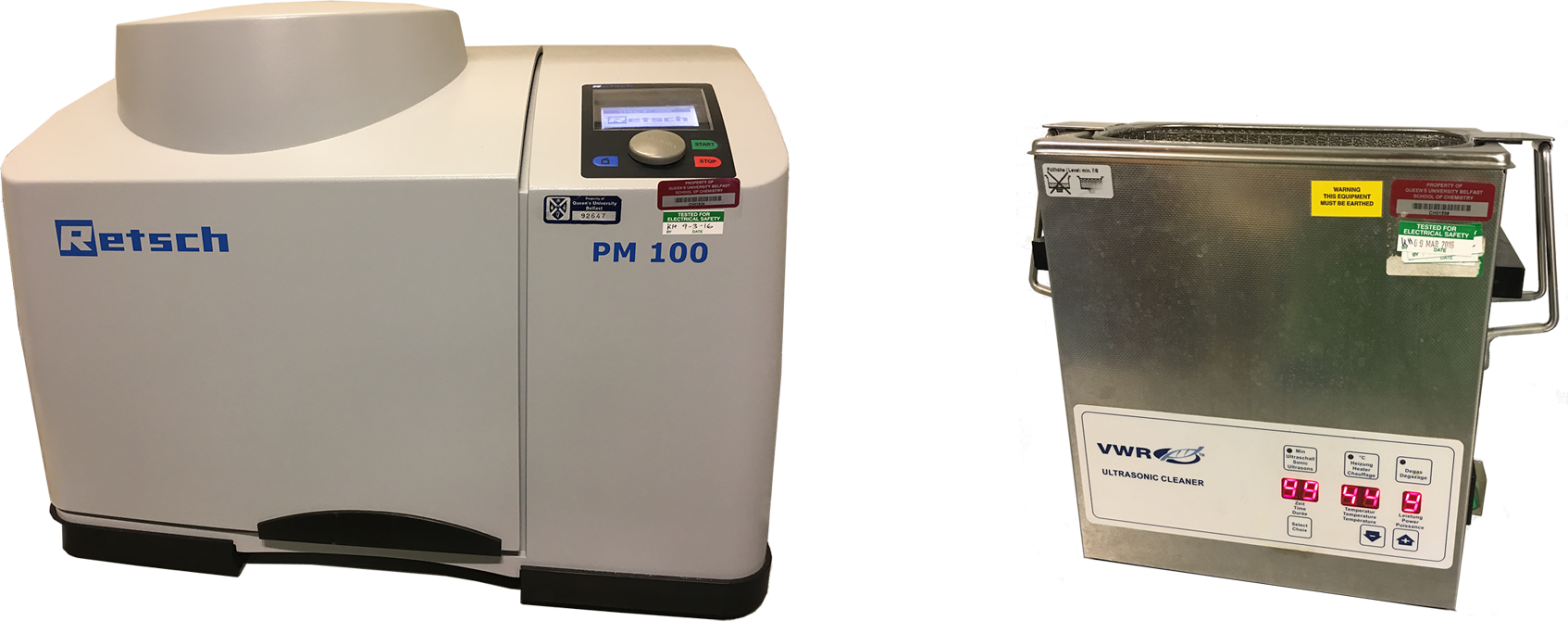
Typical laboratory employed planetary ball mill and ultrasonic bath.
